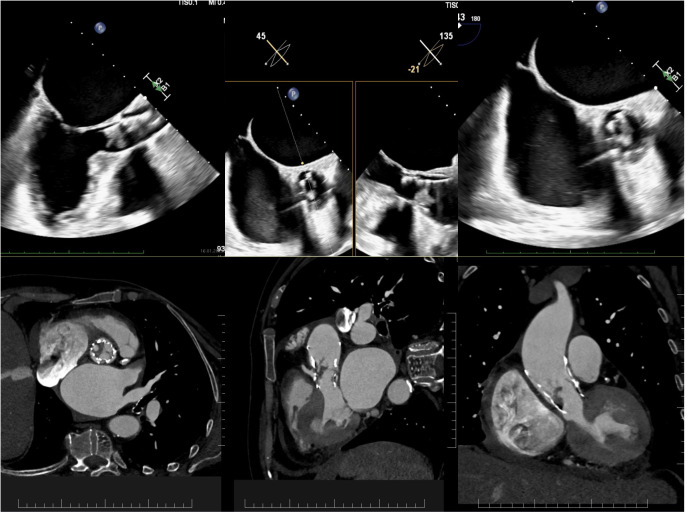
Infective endocarditis occurring three months after implantation of a 27-mm Navitor prosthesis caused by *Enterococcus faecalis*. TEE and cardiac CT demonstrated large vegetations (red arrow) and leaflet thickening (white arrows), however, the prosthetic valve was not dysfunctional, and no periprosthetic complications were identified – Supplementary Movie 1.
